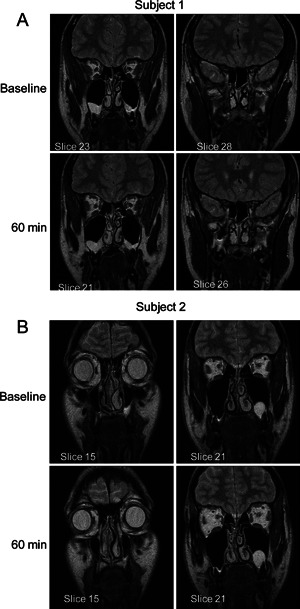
(A) MRI at baseline and at 60 min following challenge; subject 1 shows decreased airspace in both nostrils, the changes are representative for all but one of the subjects where the baseline scan showed movement artifact; (B) MRI at baseline and at 60 min following challenge; subject 2 shows unilateral changes with decreased airspace in right nostril, increased airspace in left nostril.
